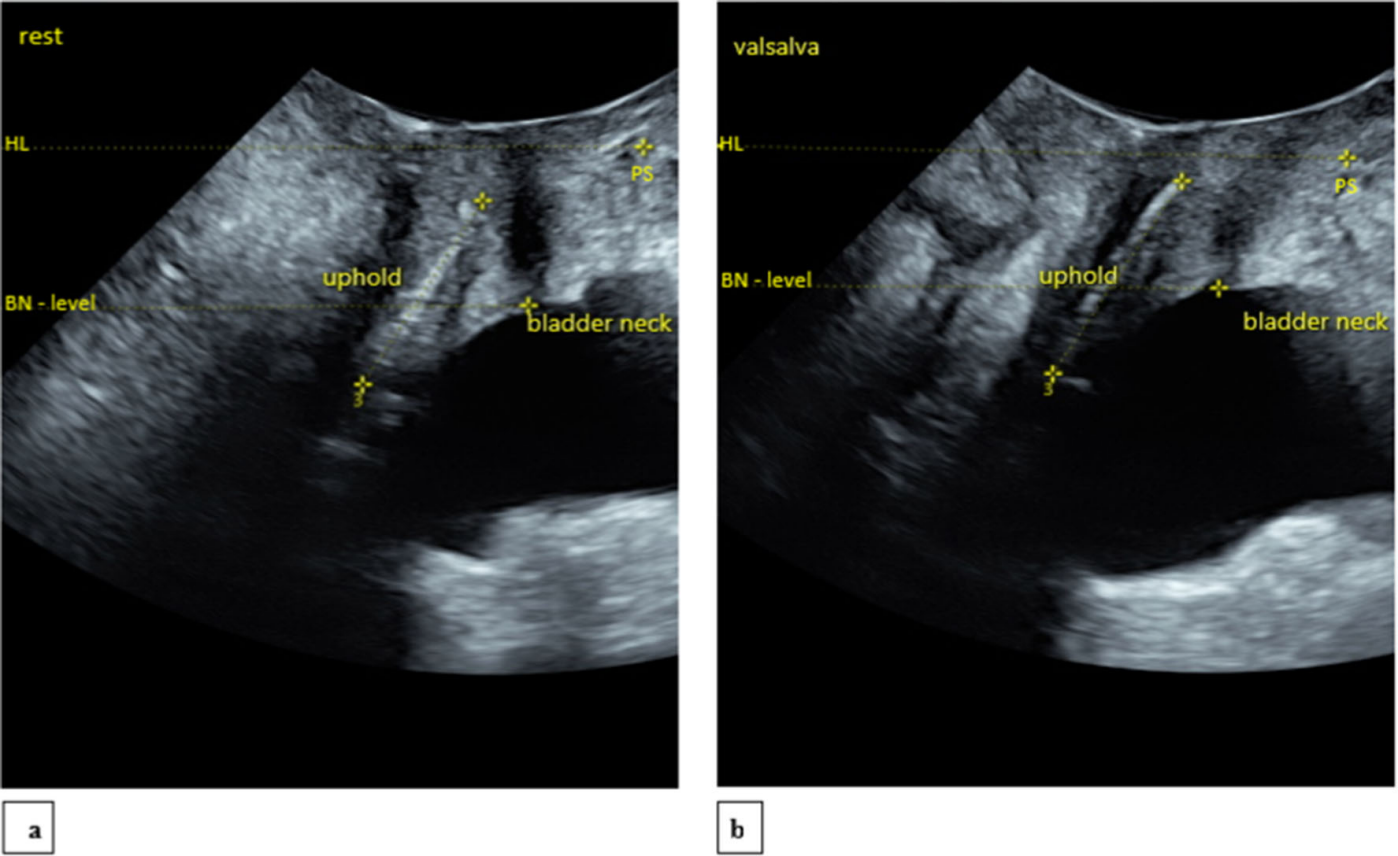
Ultrasound examination of a patient at rest (Fig. 1a) and Valsalva (Fig. 1b). HL = horizontal line, PS = pubis symphysis, BN level = bladder neck level. The total midsagittal Uphold mesh length is indicated between the upper and lower markings
